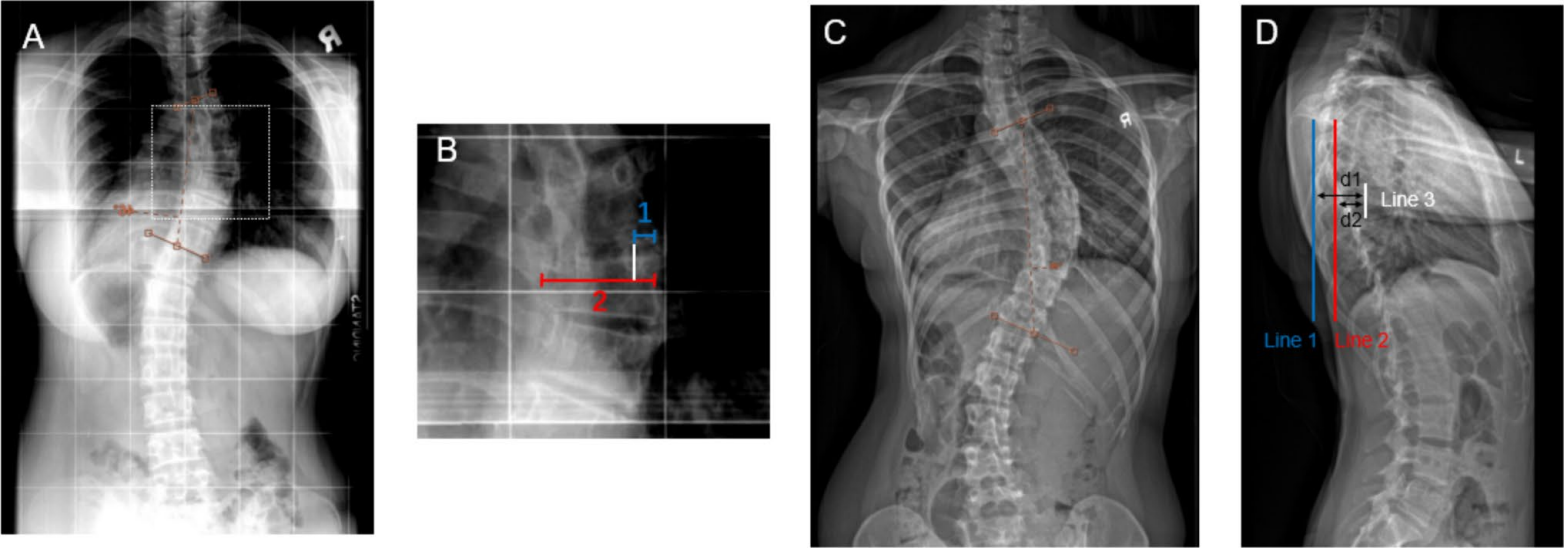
Measurement of axial rotation via modified Nash–Moe method and Rib Index. A A right thoracic curve of 46° spanning T5–T10, with the major curve apex enlarged and shown in (B). Upon (B), the axial rotation of the apical vertebra was estimated by dividing the distance from the lateral vertebral border to the central aspect of the convex pedicle shadow (1) by the width of the vertebra over the mid-vertebra (2). C, D Posteroanterior and lateral standing X-rays of a right thoracic curve of 46° spanning T5–T12. D Line 1 corresponds to a vertical tangential line to the most posterior rib point, while Line 2 corresponds to a vertical tangential line to the least projected rib point at the same height. Line 3 is a vertical line drawn against the posterior vertebral margin at a height corresponding to prior rib point measurements. The distance between Line 3 and Line 1 (d1) is divided by the distance between Line 2 and Line 3 (d2) to obtain the Rib Index
